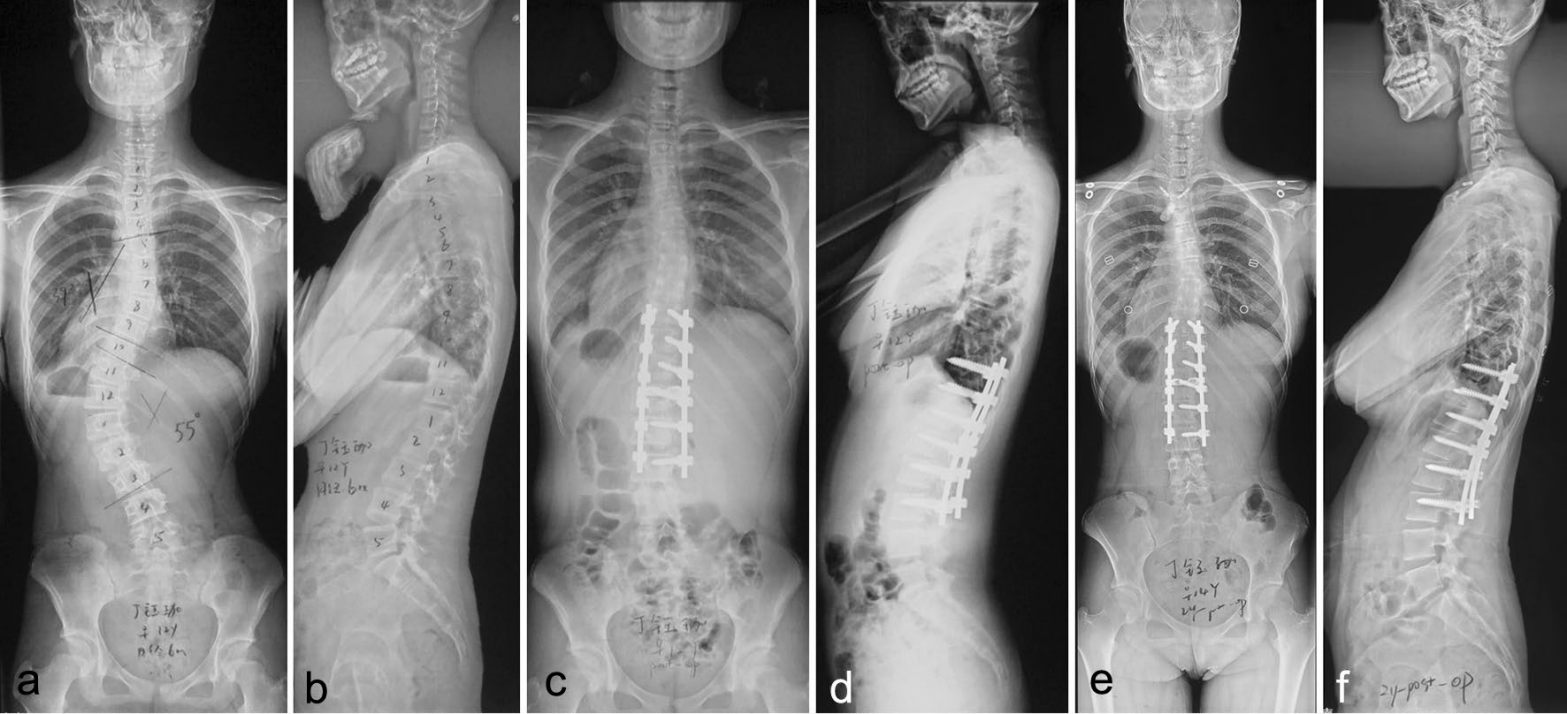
A female patient aged 12 years with 55° left thoracolumbar curve (a, b), receiving T10-L3 posterior correction surgery (c, d); 2 year postoperative radiographs did not show significant correction loss (e, f)
